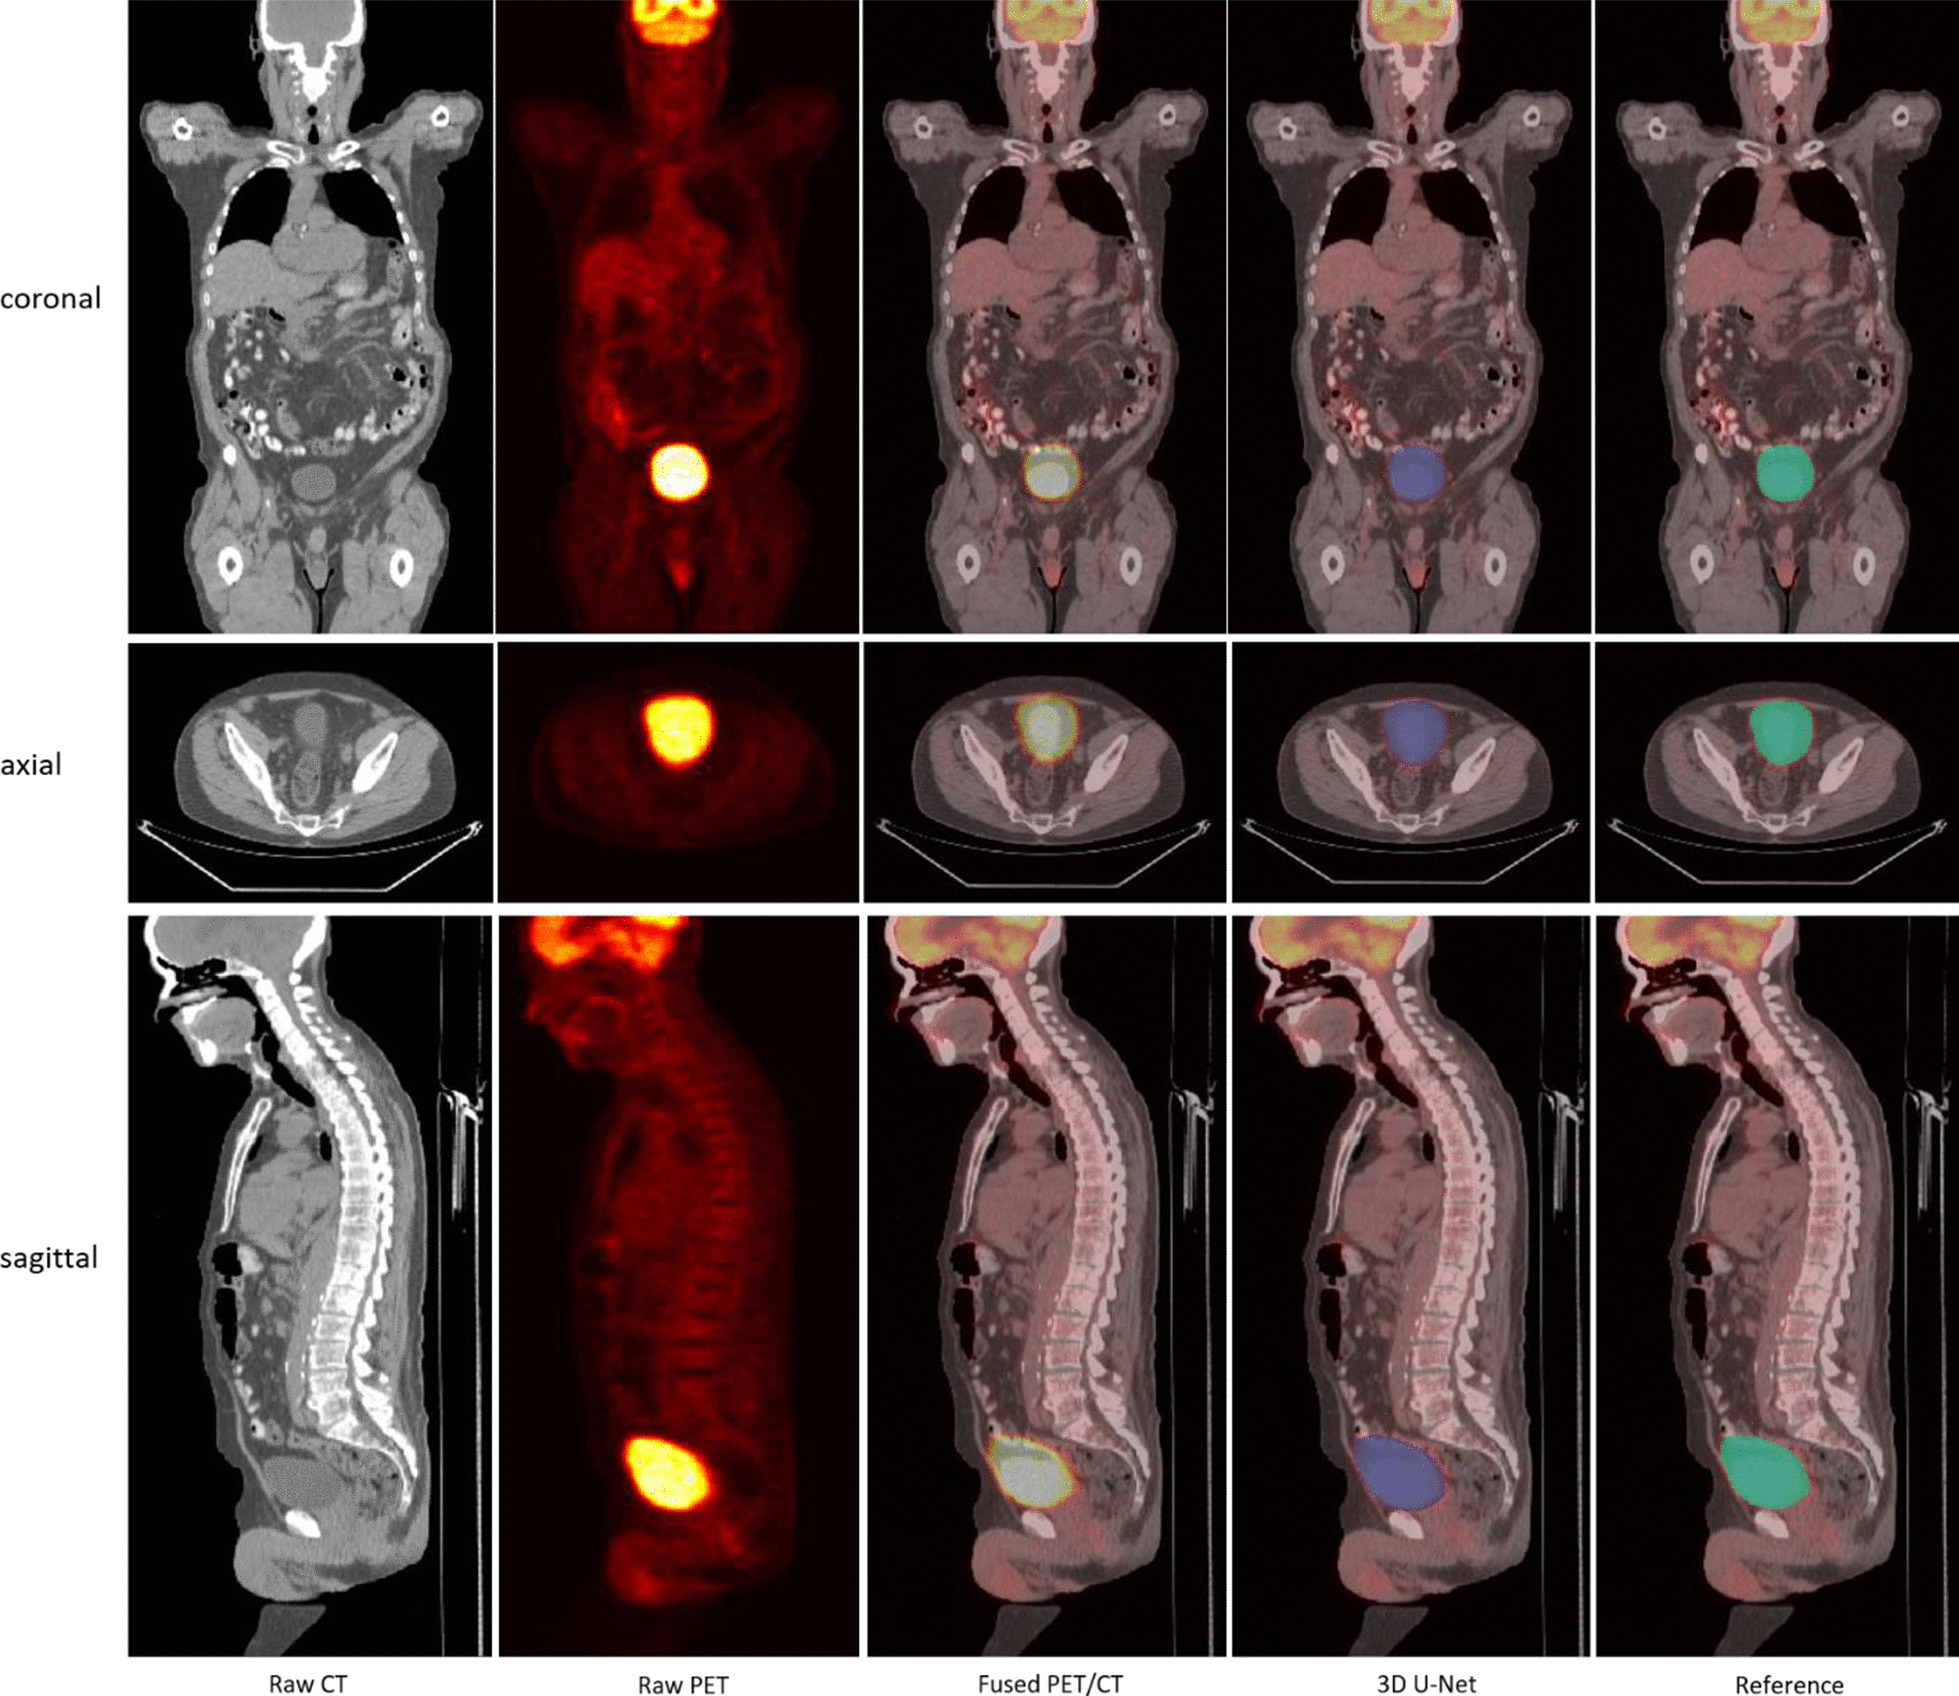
Example of a large bladder and it has a bigger bladder on PET than the one on CT. Automated segmentation is shown in purple and reference segmentation in cyan
